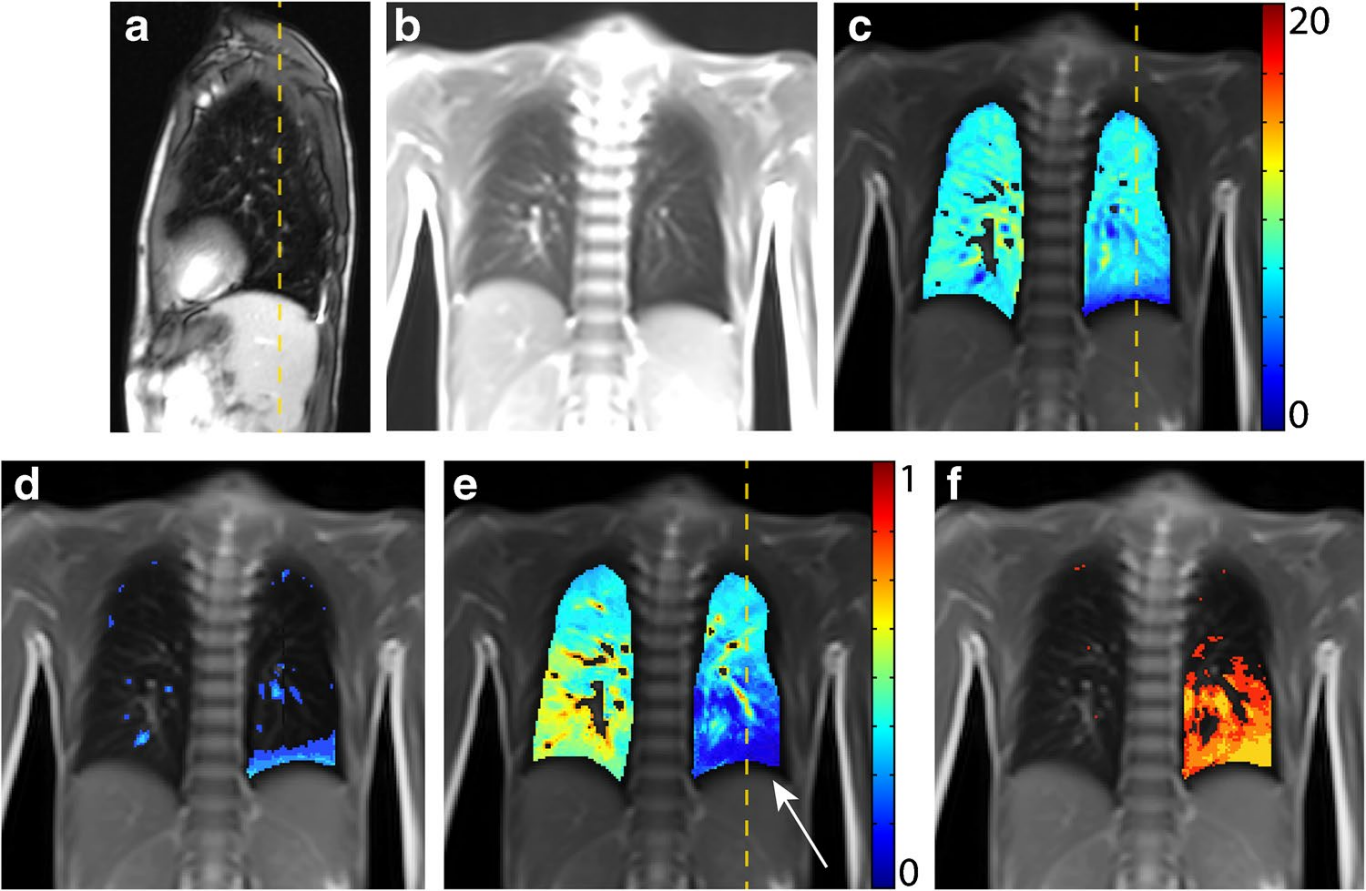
A 6-year-old boy following lobectomy of left lower lobe for CPAM type 1 with a normal lung clearance index of 7.32 turnovers. A sagittal T2-weighted image (a) (repetition time [TR]/echo time [TE] 3.3/1.3 ms) and (b) coronal T1/2-weighted image (TR/TE 1.5/07 ms) demonstrate a smaller volume of left lung and an expansion of the remaining left upper lobe into left lower thoracic cavity. c-f Coronal functional magnetic resonance imaging maps: (c) fractional ventilation, (d) fractional ventilation defect percentage (brighter blue areas represent reduced function), (e) relative perfusion and (f) relative perfusion defect maps (brighter orange areas represent reduced function) show a fractional ventilation defect percentage (13.4%) and a perfusion defect percentage (18.3%) within the norm. However, in the area of the former left lower lobe, which is now replaced by the upper left lobe, a locally reduced relative perfusion is visible in (e) (*white arrow*). *Yellow dashed lines* indicate plane levels. *CPAM* congenital pulmonary airway malformation
